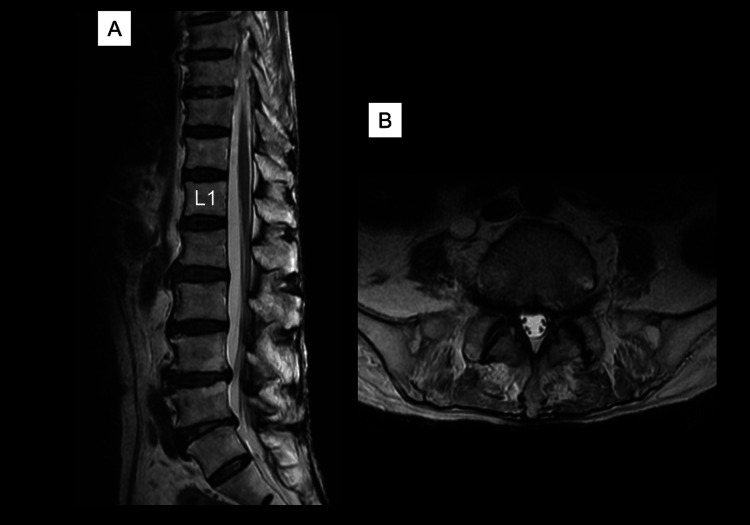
T2-weighted lumbar MRI of the patient (A) Sagittal section and (B) horizontal section (L5/S1). Spinal canal stenosis is observed.
